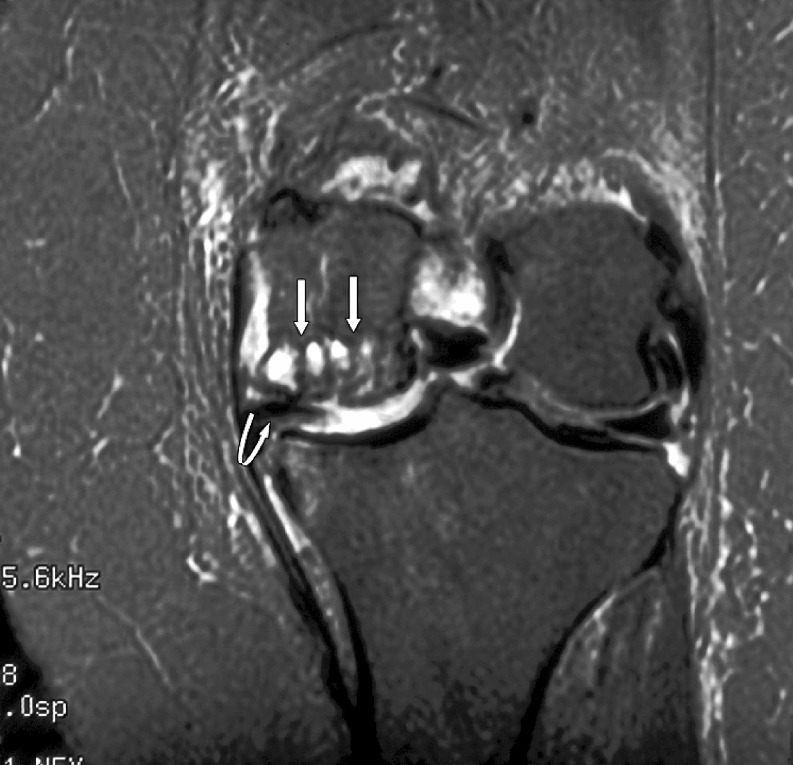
Cystic pattern with a total knee joint replacement in an 82-year-old female patient. The radiograph demonstrated subchondral lucency consistent with a geode and irregularity of the adjacent subchondral bone plate. Coronal fast fat-suppressed T2-weighted image (TR 3000/TE 56/256 × 192) shows complete loss of hyaline cartilage on the medial femoral condyle and adjacent moderate subchondral cystic areas consistent with geodes, and the adjacent bone marrow edema (*arrows*). Note also the extruded body of the medial meniscus containing a degenerative horizontal cleavage tear (*curved arrow*)
